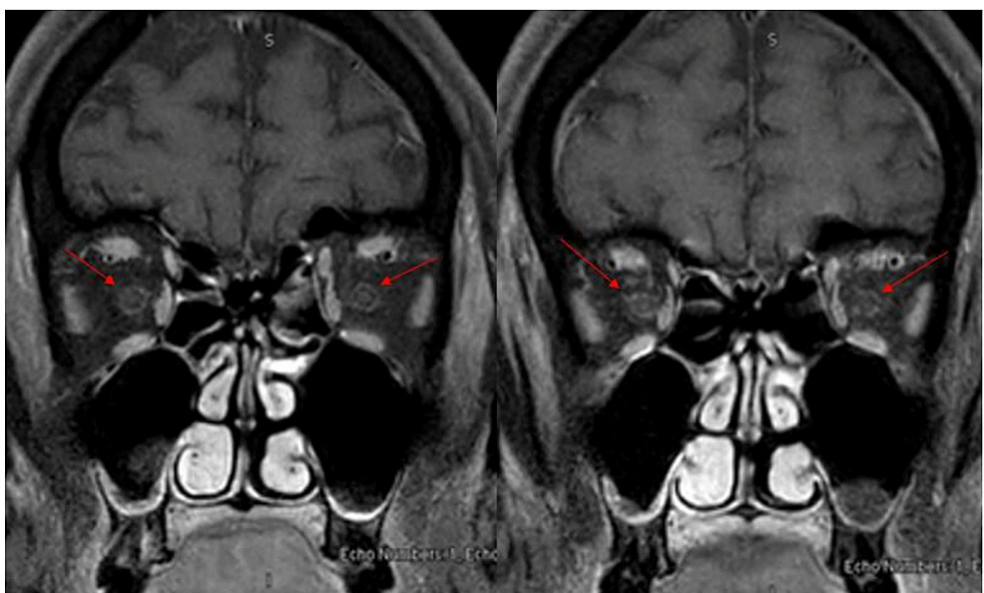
MRI orbits T1-weighted image with gadolinium MRI orbits T1-weighted image with gadolinium fat-suppressed coronal view showed distal intra-orbital optic nerve sheath faint enhancement and appearance of doughnuts like shape (as shown by red arrows).
